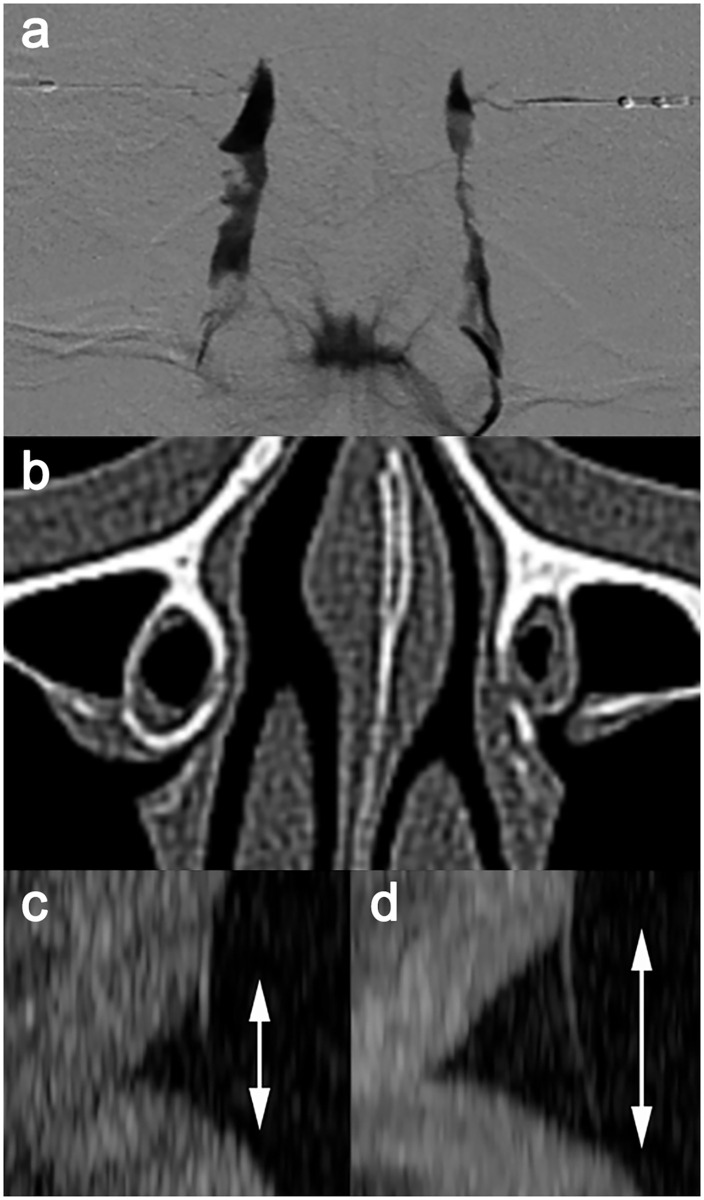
Measuring the bony nasolacrimal duct (NLD) size of a patient showed unilateral success after bilateral nasolacrimal silicone intubation. (a) Preoperative dacryocystography shows a dilated NLD in the right eye and stenosis of the sac-NLD junction in the left eye. (b) A preoperative computed tomography axial image shows that the smallest minor axis diameter of the right eye was larger than that of the left eye (white lines, 5.6 mm vs. 4.2 mm). (c, d) Anterior segment optical coherence tomography shows tear meniscus between the cornea and lower eyelid; tear meniscus height (white arrow) is normal in the right eye (298 μm) and high in the left eye (428 μm) at 8 months after surgery.
